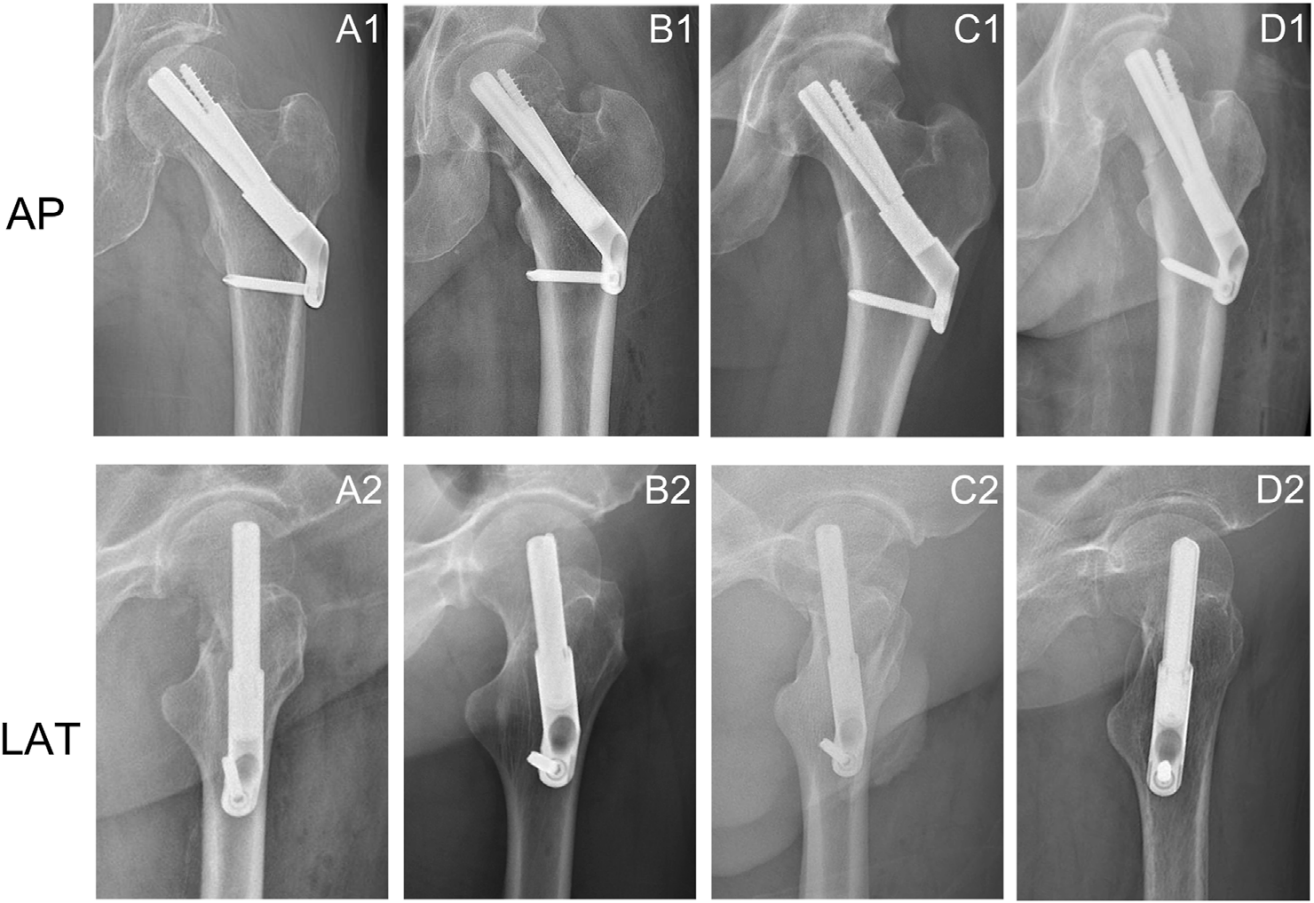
Anteroposterior and lateral X-rays of femoral neck fractures treated with FNS showed changes in the position of the FNS. (A1, A2) The bolt was implanted along the axis of the femoral neck in the anteroposterior and lateral views; (A2, B2) Change in bolt length; (C1, C2) Downward movement of the bolt in the anteroposterior view and posterior rotation of the bolt in the lateral view; (D1, D2) External rotation of the bolt in the anteroposterior views and anterior rotation of the bolt in the lateral view.
